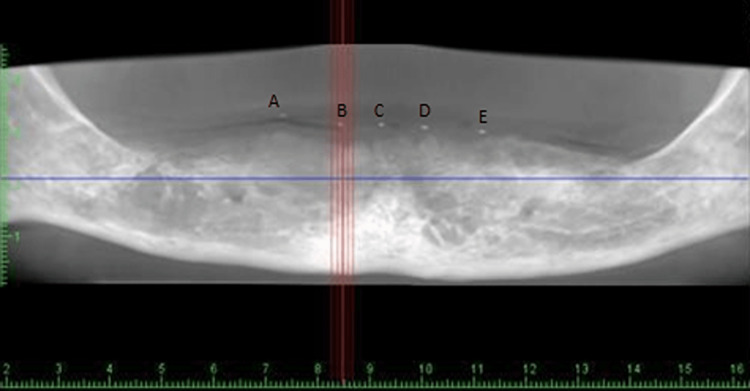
CBCT scan of the edentulous mandible after placing the stent with embedded gutta-percha cones at prospective implant sites. With the assistance of radio-opaque gutta-percha cones, one can clearly visualise the area of the Misch prospective implant sites A, B, C, D, and E on the CBCT scan. CBCT: cone beam computed tomography.
